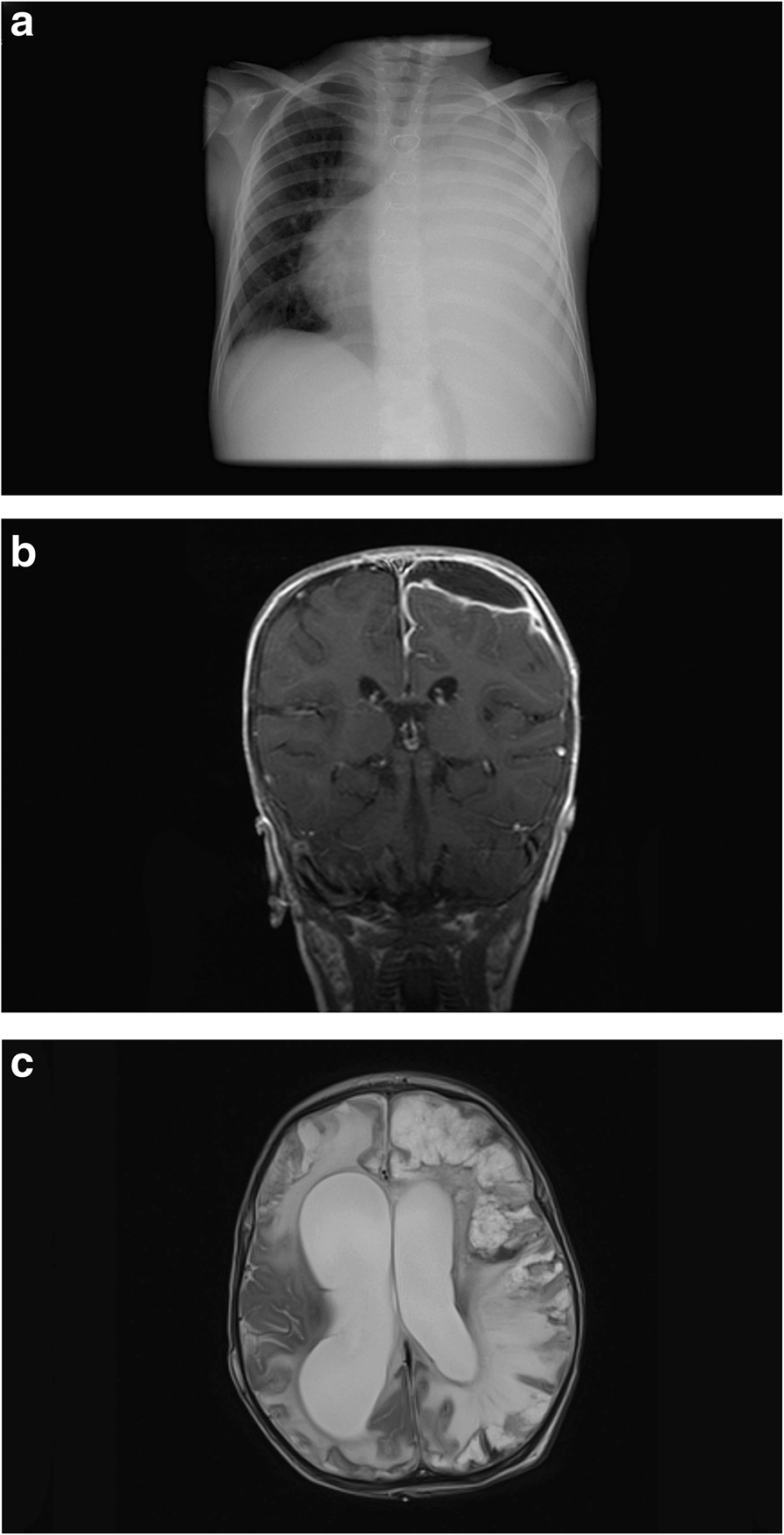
Radiological findings in three patients with invasive pneumococcal disease. a: Chest X-ray: Widespread, significant opacification of left hemithorax representing lobar pneumonia with effusion secondary to *S. pneumoniae* in a six year old girl. b: Coronary T1-weighted MRI after contrast agent injection: Subdural hygroma in the left hemisphere and increased meningeal enhancement in a one year old boy diagnosed with meningitis secondary to *S. pneumoniae.*c: T2-weighted MRI without contrast agent: Internal hydrocephalus and pronounced necrosis of the cerebral parenchyma (left > right) in a one month old girl, secondary to IPD with fatal outcome.
